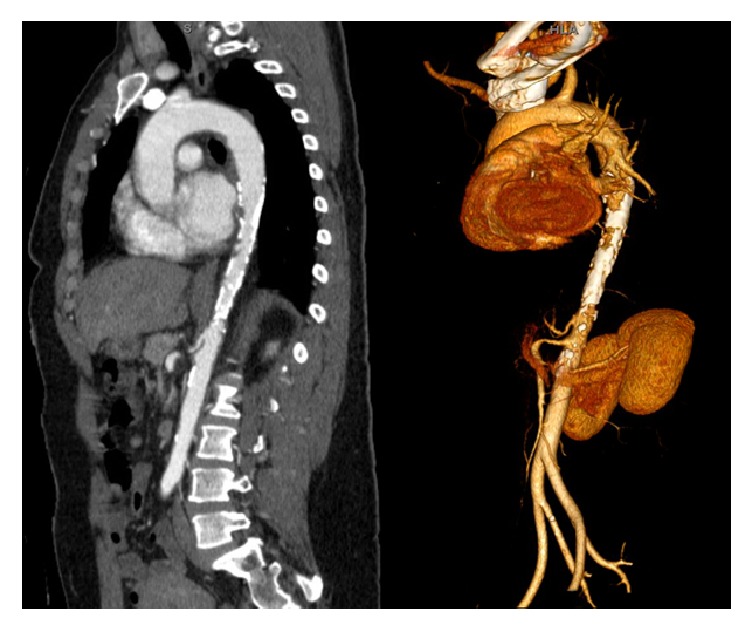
CTA of showing the area of stenosis in the supraceliac aorta with 3D reconstruction.
